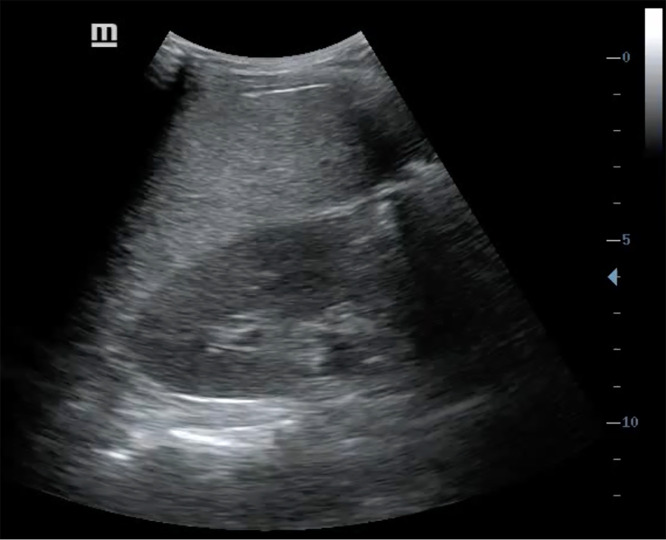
Ultrasound of the right upper quadrant revealed a diffusely hyperechoic “bright liver” pattern without focal lesions.
